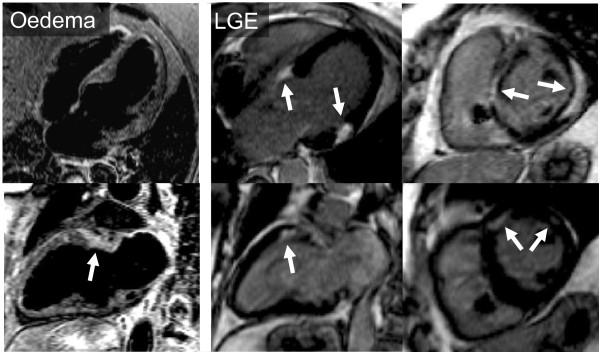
Cardiac MRI with T2-weighted STIR images (left panel) showing oedema (arrow) and (middle and right panels) extensive patchy late gadolinium enhancement typical of sarcoid.
